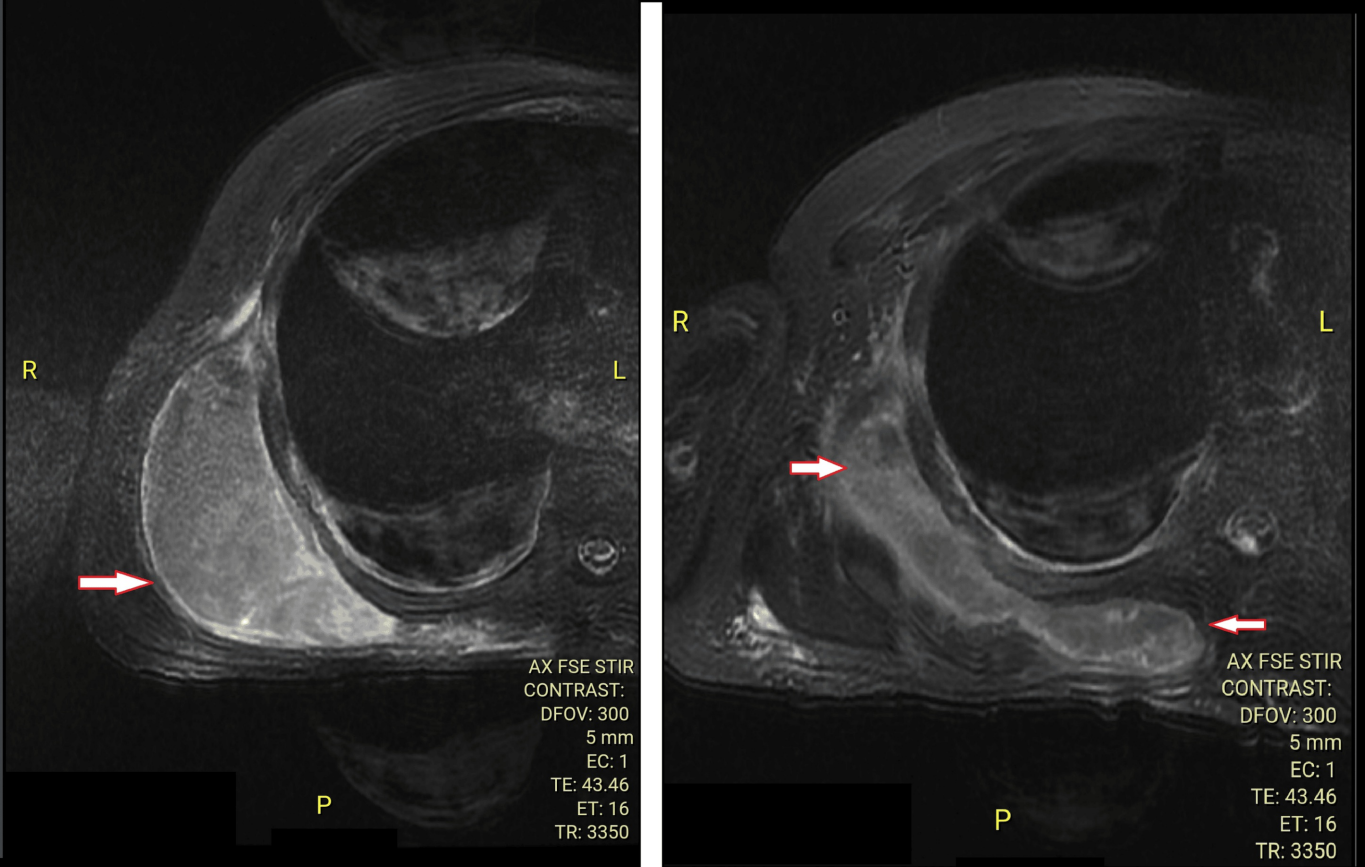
MRI chest with contrast, axial view, showing the subscapular abscess.
